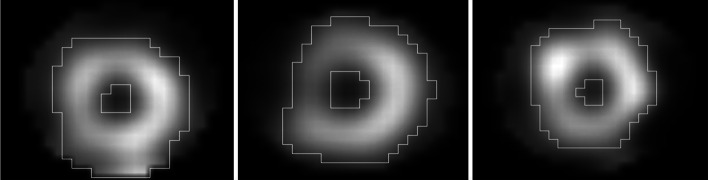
Myocardial ROI in the uncorrected clinical SPECT images of three patients. The ROI was defined as the pixels whose counts exceeded 45% of the maximal pixel value
